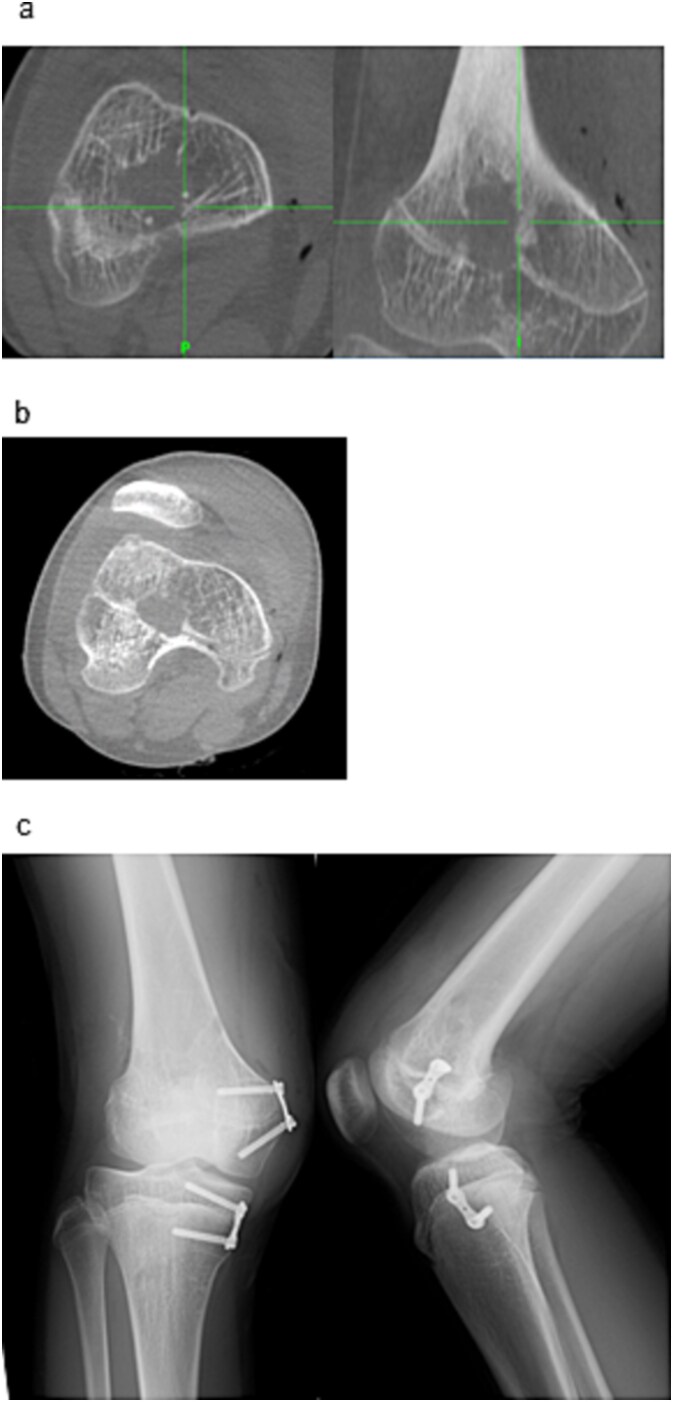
Post-resection imaging and implant placement. (a) Post-resection CT confirming complete removal of the physeal bar. (b) No evidence of cortical perforation was seen. (c) Postoperative radiographs (anteroposterior and lateral) showing placement of the eight-plate across the distal medial femur and proximal medial tibia.
